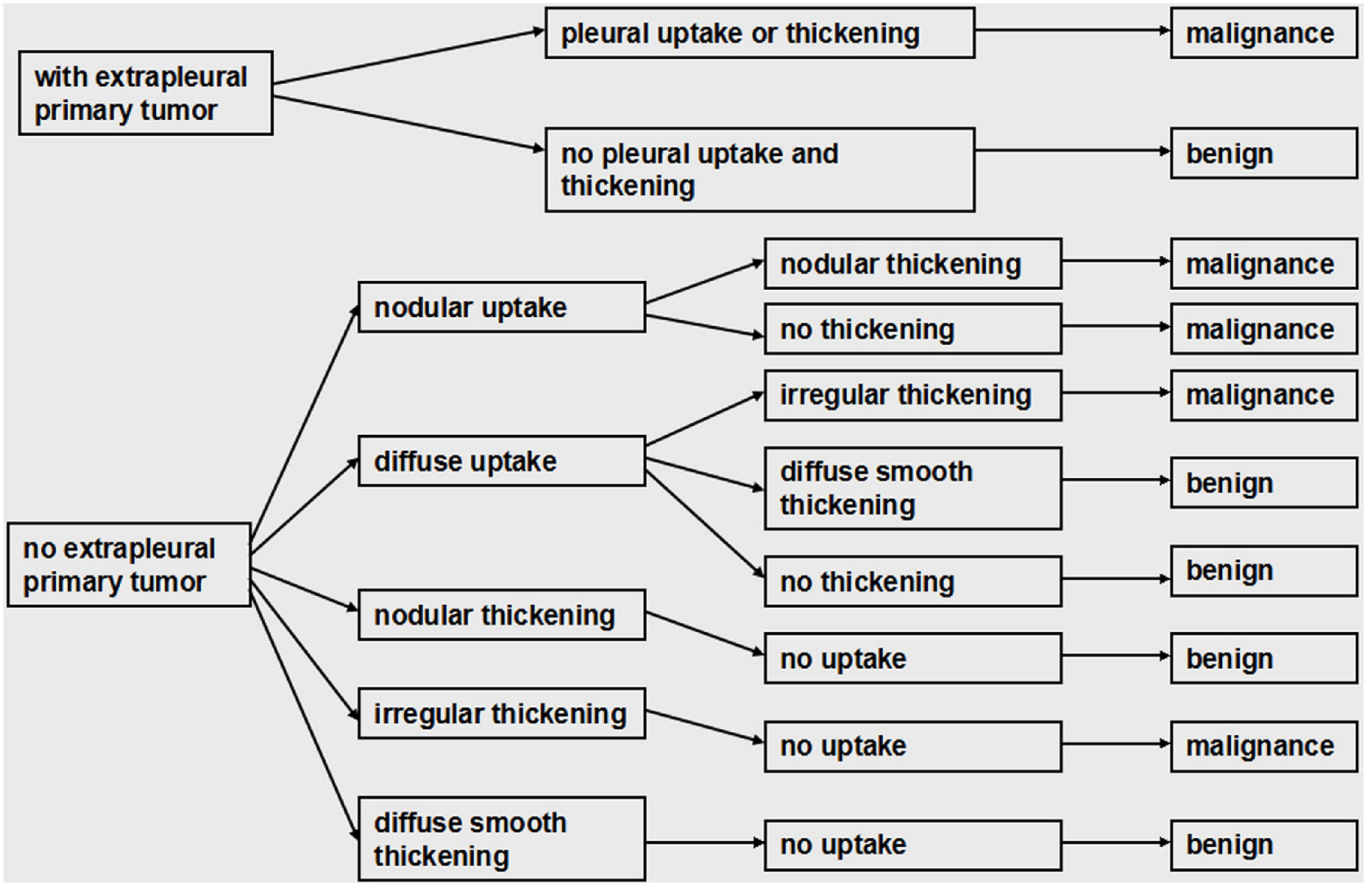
The diagnostic criteria of ^18^F-FDG PET/CT integrated imaging in the differential diagnosis of pleural effusion.
